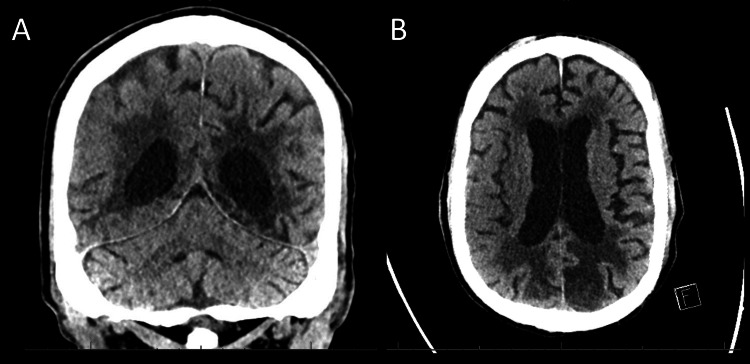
Head CT without contrast. Noted left and right chronic infarcts. (A) Demonstrate coronal view. (B) Demonstrate axial view.
